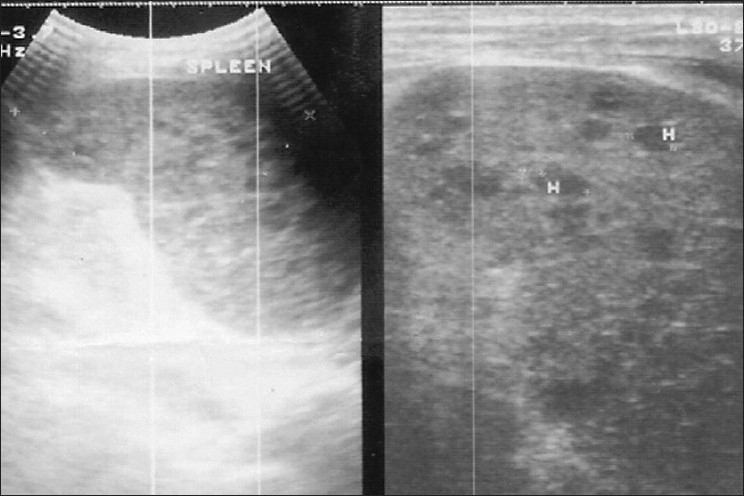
Ultrasound abdomen showing multiple hypoechoic lesions in the spleen
